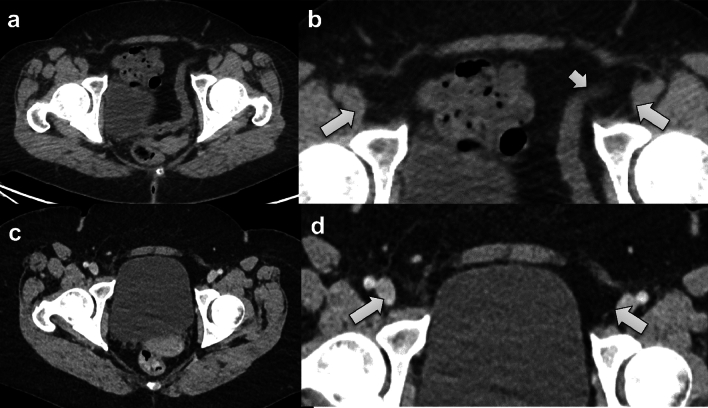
50-year-old female patient with prior Roux-en-Y gastric bypass and bilateral femoral hernias. Representative Valsalva CT image (A) and corresponding magnified image of the groin (B) shows bilateral femoral hernias (long arrows in B) with a protruding bowel loop on the left side (short arrow in B). A non-Valsalva CT scan (C, and magnified image of the groin in D) done for abdominal pain 3 months prior does not depict the right-sided femoral hernia clearly whereas the left-sided hernia is less conspicuous, and the protrusion of bowel is not depicted
